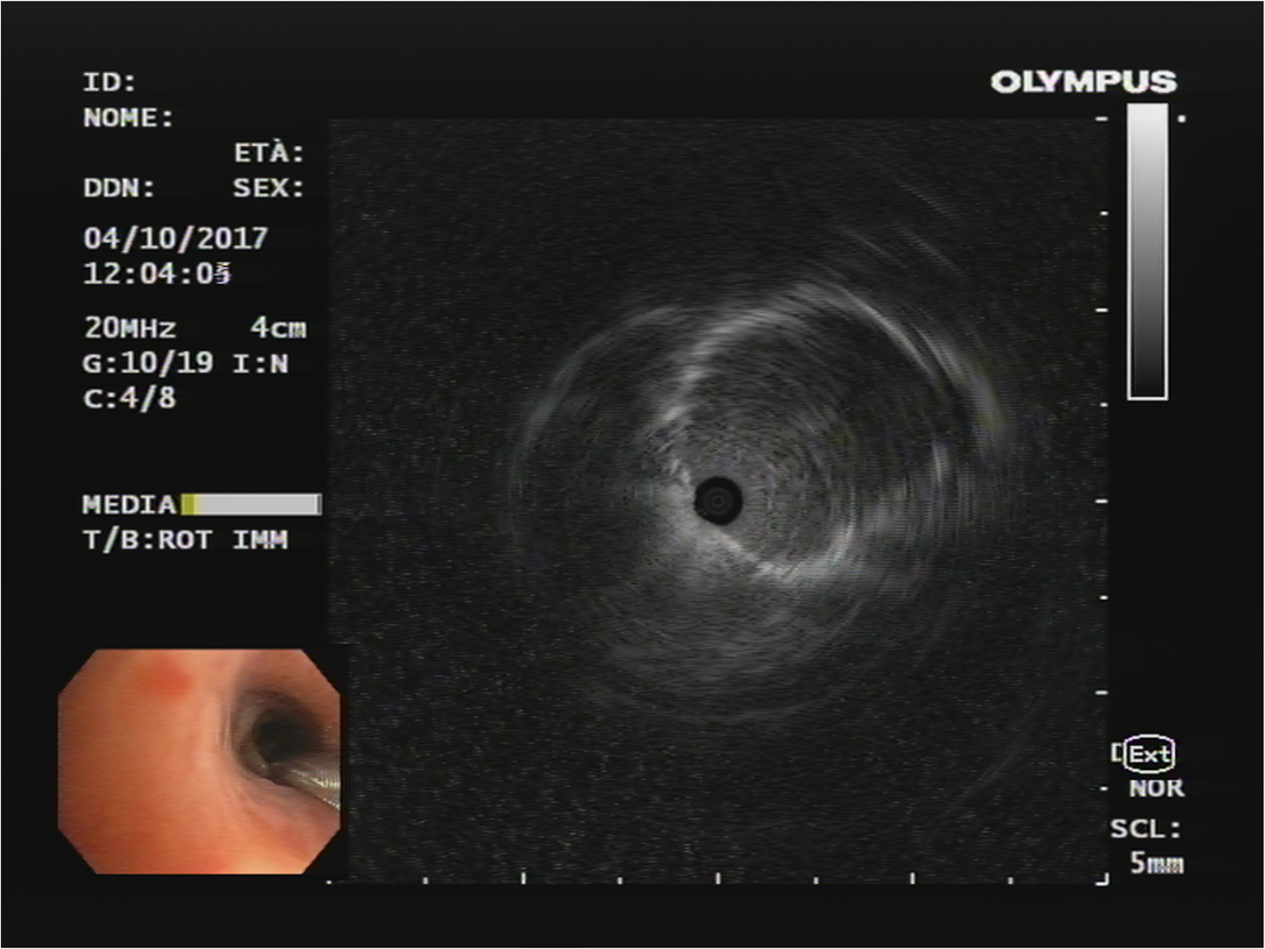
Radial probe endobronchial ultrasound image in LB3a bronchus indicating the presence of a hypoechogenic nodule from 1 ‘clock to 3 o’clock
